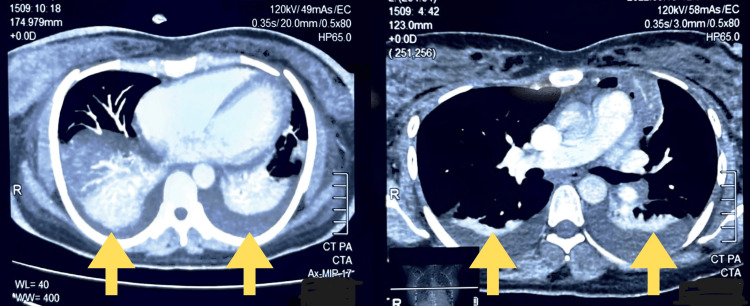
High-resolution computed tomography (HRCT) showing bilateral multifocal areas of dense patches of ground glass opacities with surrounding consolidative changes and moderate pleural effusion and left-sided cavitatory lesions. Keeping in view the patient’s history of dengue hemorrhagic fever, these findings are suggestive of pulmonary hemorrhage with superadded bacterial pneumonia. Yellow arrows point to pleural effusion.
